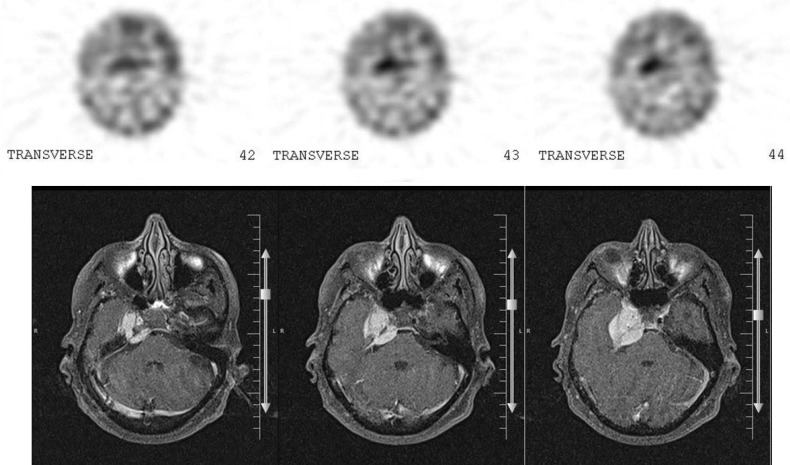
Reconstructed ^99m^Tc-TRODAT images as well as the corresponding MRI (T2-weighted) which show the tracer uptake in the meningioma (MRI and SPECT images both were presented parallel to the orbito-meatal line).
